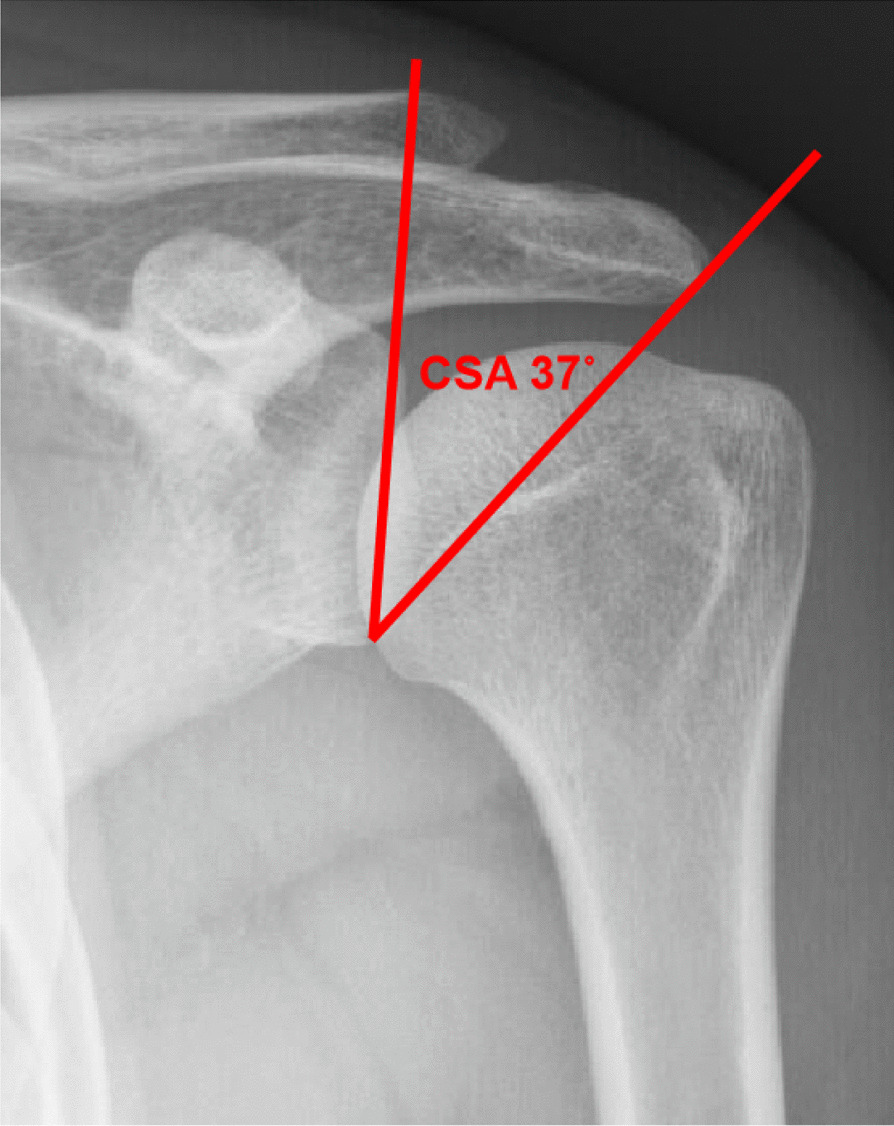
The critical shoulder angle (CSA) measured on true anteroposterior radiographs
